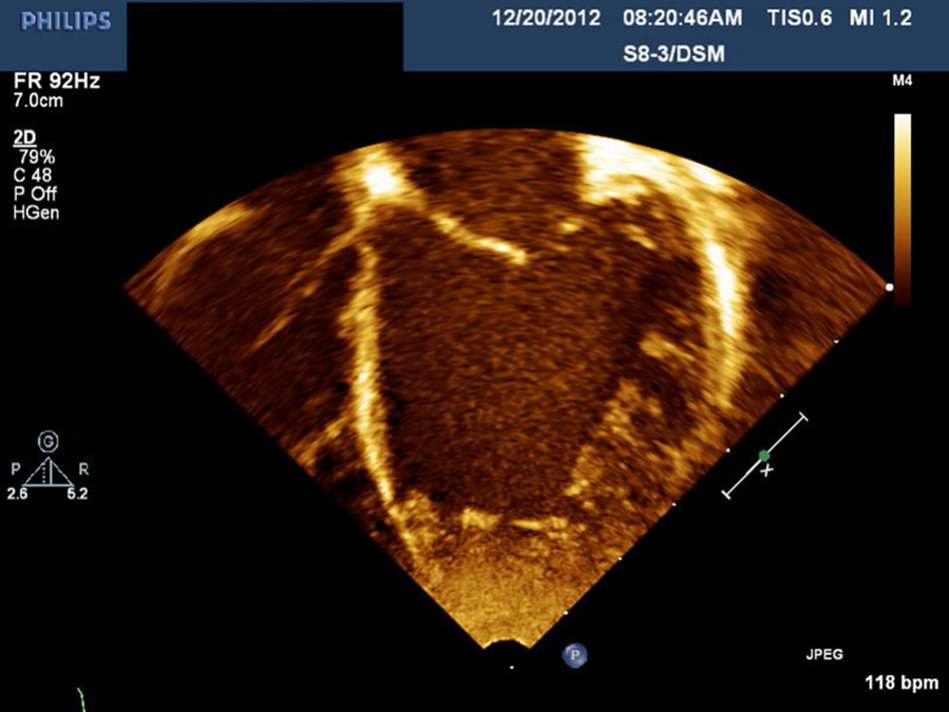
Echocardiogram (apical 4-chamber view) of patient with BTHS depicting LVNC with associated DCM phenotype. Note the deep LV trabeculations and dilated left ventricular chamber.
